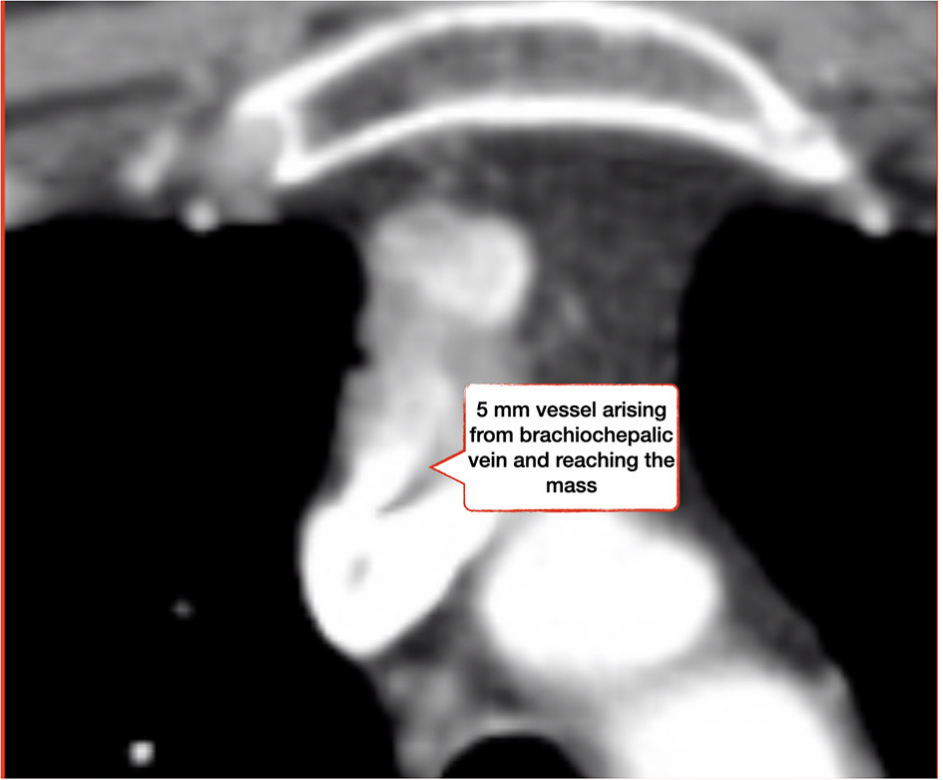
CT scan showing a 5 mm vessel arising from left brachiocephalic vein and reaching the mediastinal mass.
